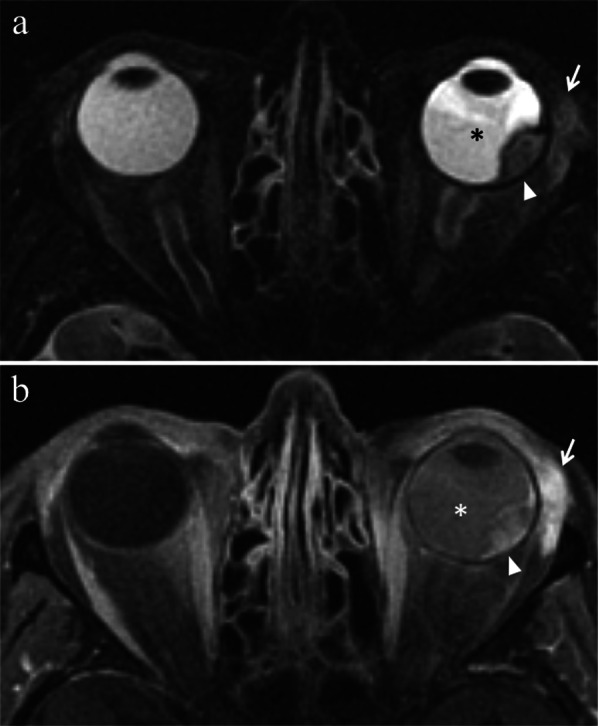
A 75-year-old woman with inflammatory swelling of the lacrimal gland after proton-beam radiotherapy for choroidal melanoma of the left eye. The same patient as in Fig. 12. Axial a T2-weighted turbo spin-echo STIR and b contrast-enhanced fat-suppressed T1-weighted images demonstrate an enlarged left lacrimal gland with marked enhancement (white arrows). A dome-shaped intraocular lesion (white arrowheads) is recognizable along the lateral aspect (temporal area) of left globe, adjacent to the lacrimal gland. Note the inhomogeneous signal intensity of the left vitreous body on T2- and T1-weighted sequences (asterisks), a finding consistent with vitreous hemorrhage
